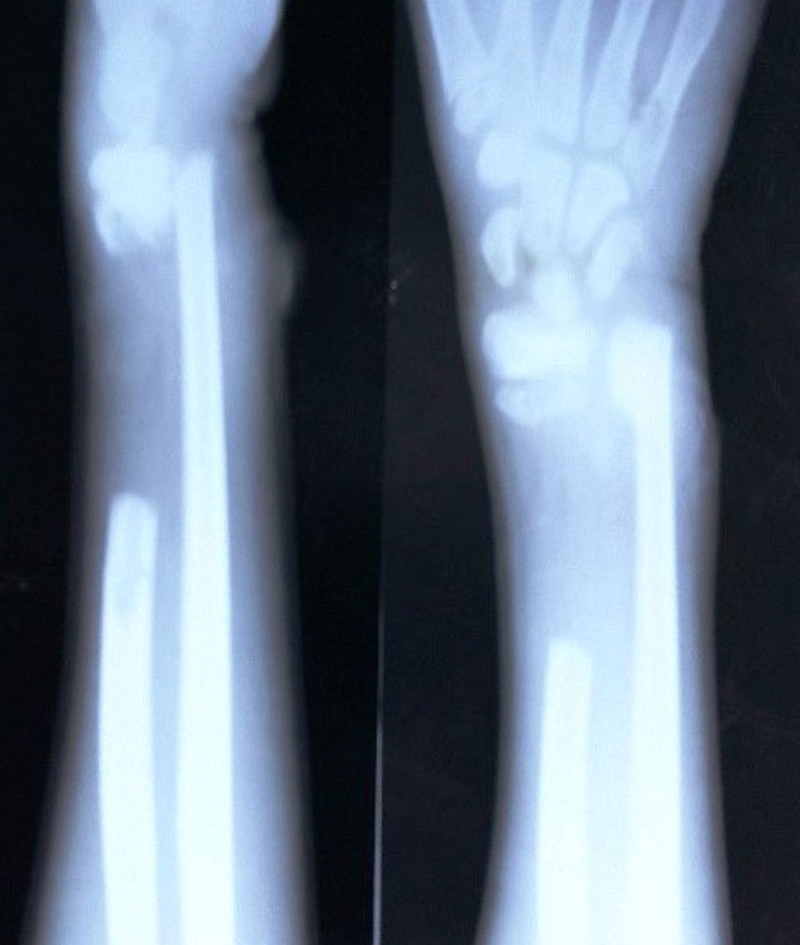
Anteroposterior and lateral X-rays of the forearm with a wrist, at initial presentation four days after injury, showing the 12-cm diaphyseal bone defect of the radius.
